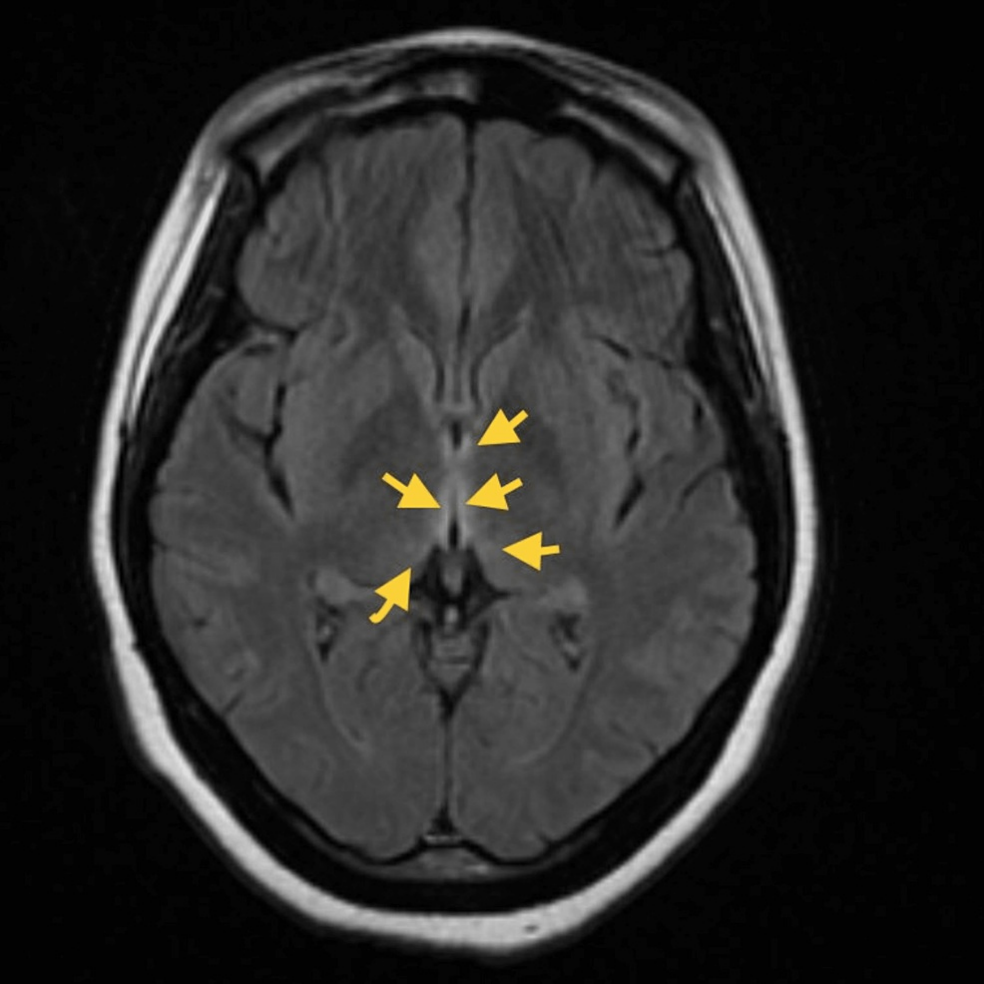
MRI of the brain without contrast FLAIR sequence MRI showing a T2 shine-through with mild edema along the hippocampus, mammillary bodies, periaqueductal gray matter, medial thalamus, and hypothalamus consistent with WE. MRI, magnetic resonance imaging; FLAIR, fluid-attenuated inversion recovery
